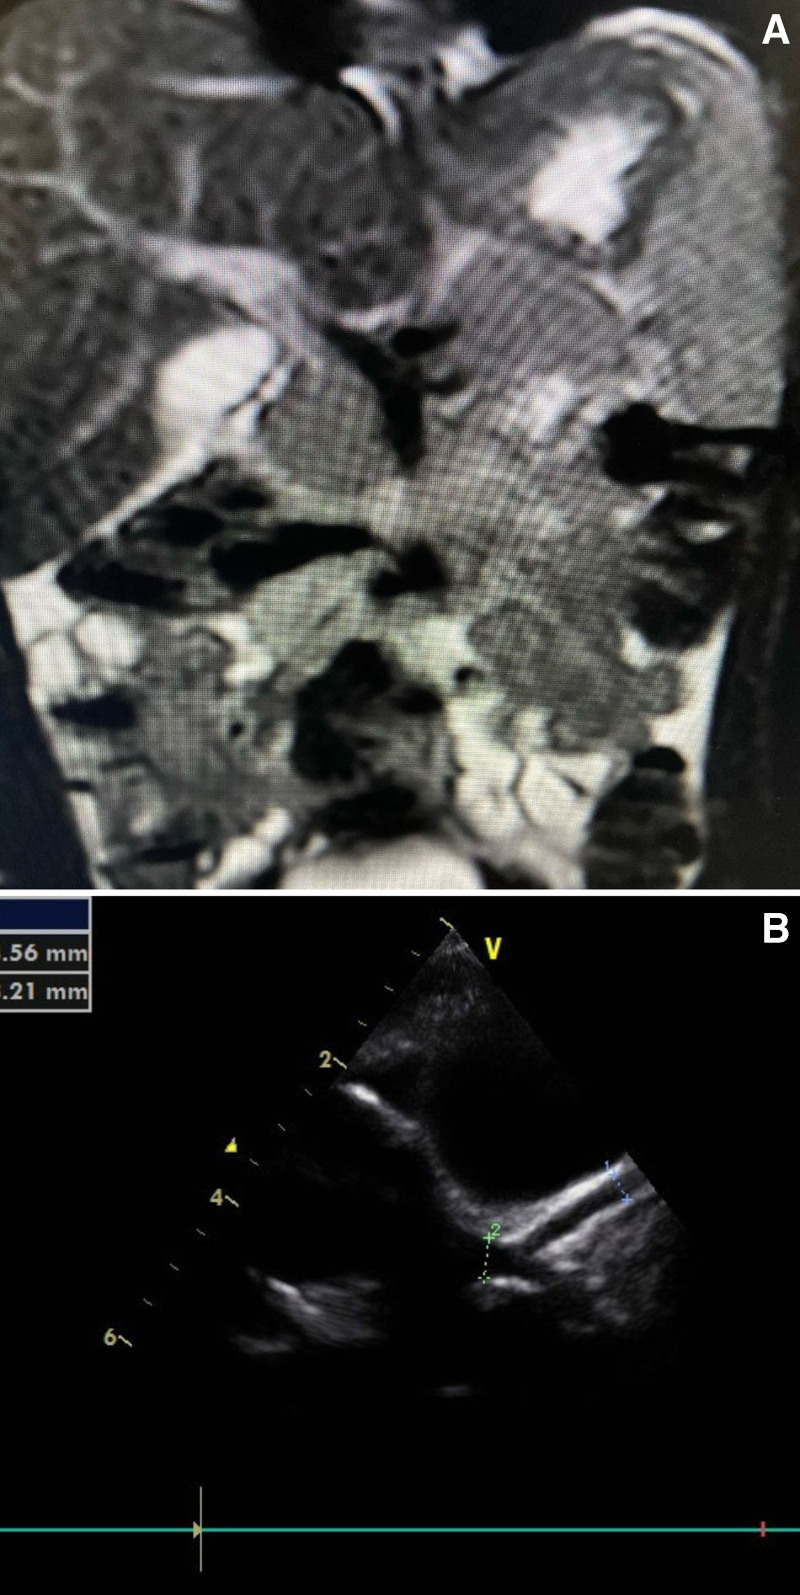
A) MRCP showing bulky pancreas and fat stranding around tail. B) Echocardiography showing dilated LMCA and LAD with perivascular brightness. LAD = left anterior descending artery; LMCA = left main colonary artery; MRCP = magnetic resonance cholangio pancreatography.
